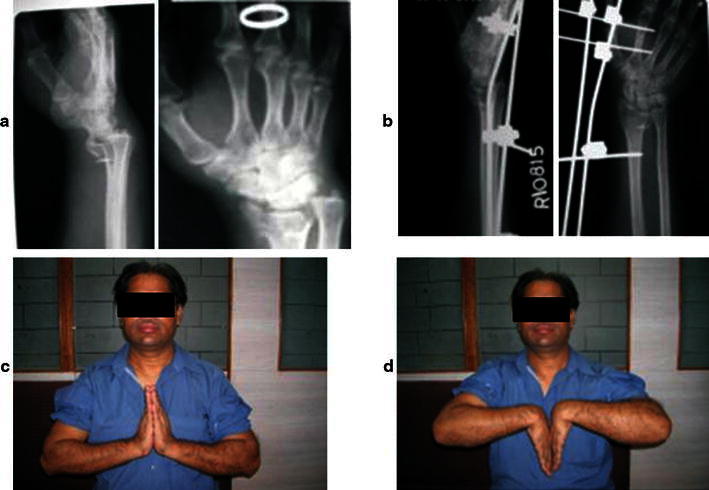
Pre- and postoperative radiographic image of the wrist of a patient in external fixation (a,b). Clinical picture of a patient treated with external fixation after 1 year follow-up (c, d)
